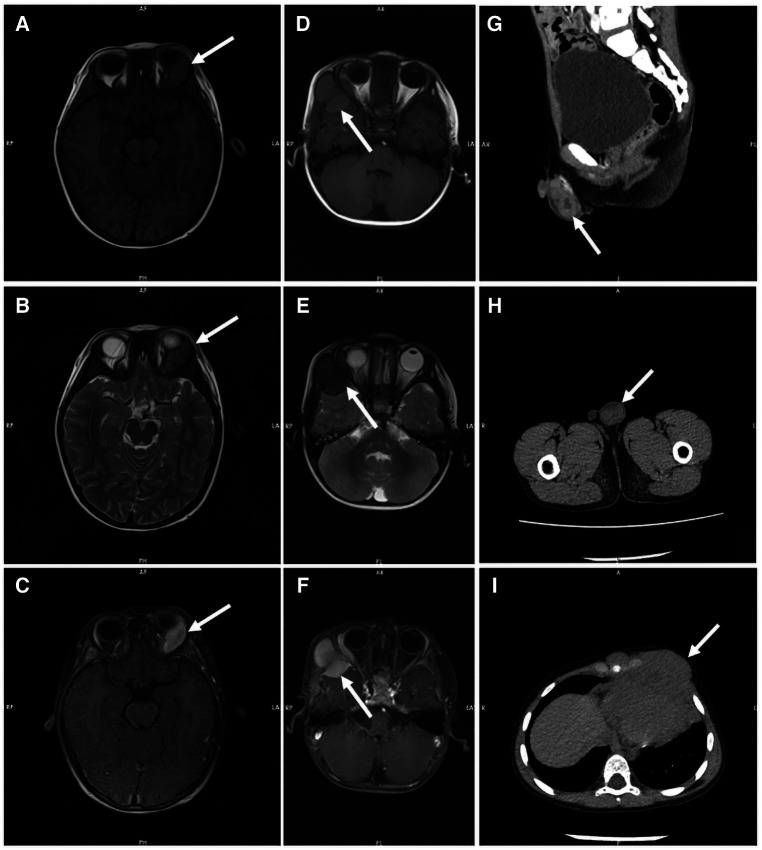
Radiological data on part patients. Both T1-weighted MR images (A) and T2-weighted MR images (B) showed equal signal intensity in the left orbital region of patient 2, which was significantly enhanced by contrast agent injection (C). T1 weighted MR images showed equal signal intensity (D), and T2 weighted MR images showed low signal intensity (E) in the frontal and temporal lobes of patient 6, with uniform enhancement and adjacent brain parenchymal compression (F). CT showed mass soft tissue density foci from the testis of patient 11 (G and H) and the mediastinum of patient 9 (I).
